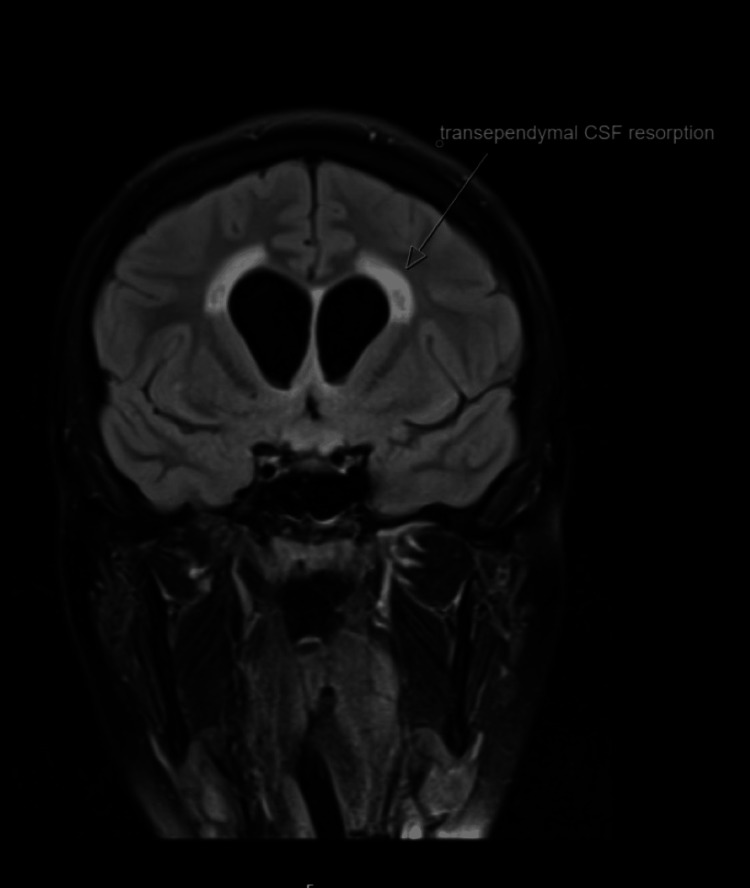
MRI of the head with contrast. White arrows: evidence of hydrocephalus.
